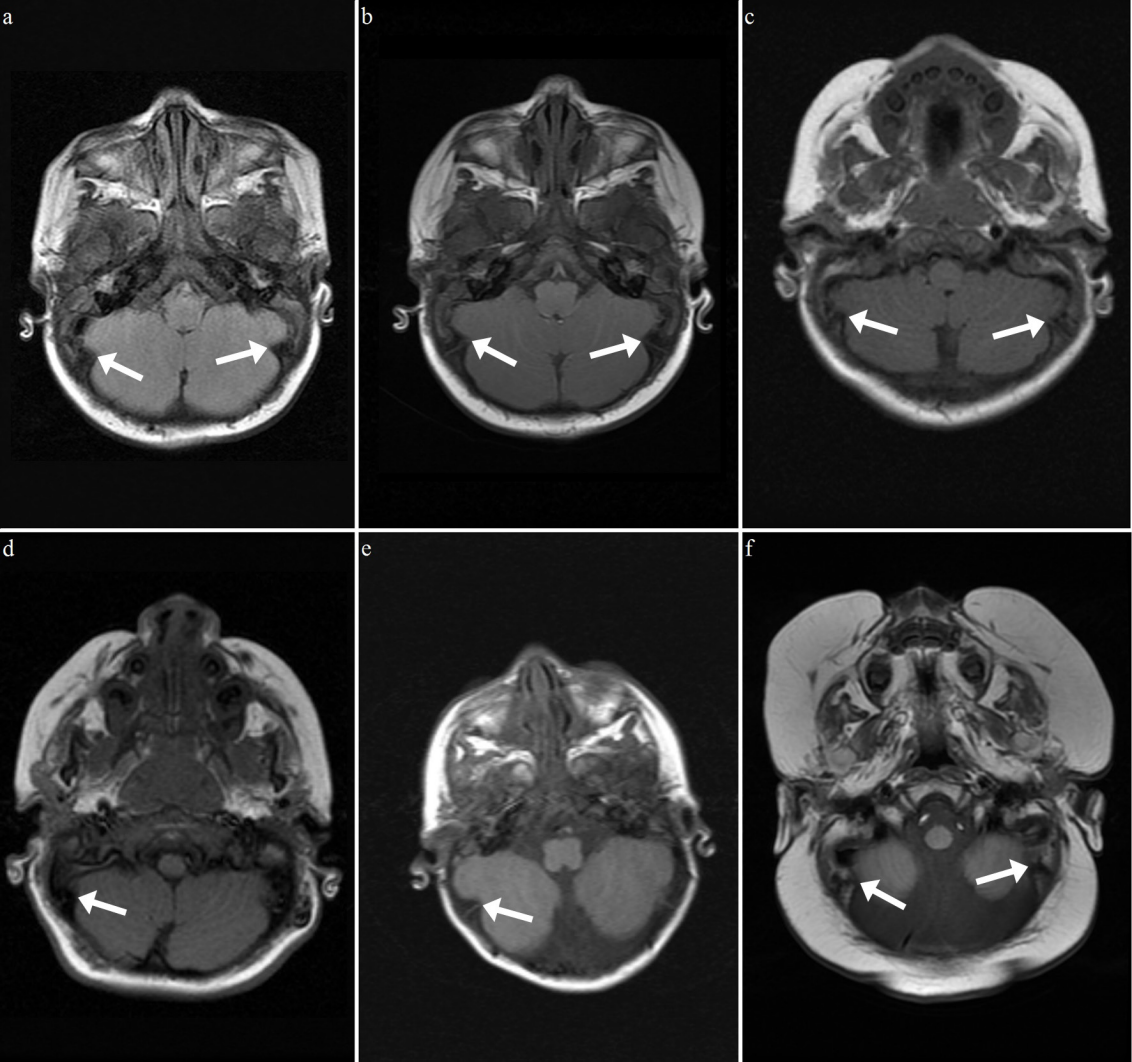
Axial FLAIR (a) and T1 weighted MR images (b–f ) show unilateral or bilateral IHOMs (white arrows) of 6 different patients with MPS type I. Note: MRIs shown in a and b belong to twin siblings. (a: 8 months old, b: 8 months old, c: 13 months old, d: 4 years old, e: 16 months old, f: 23 months old).
